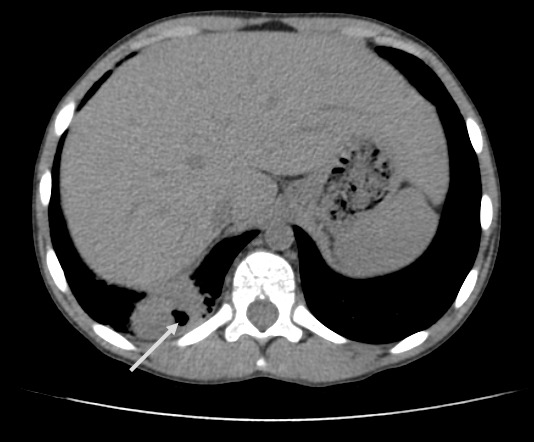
Chest computed tomography: cavitatory pneumonia of right lower lobe
